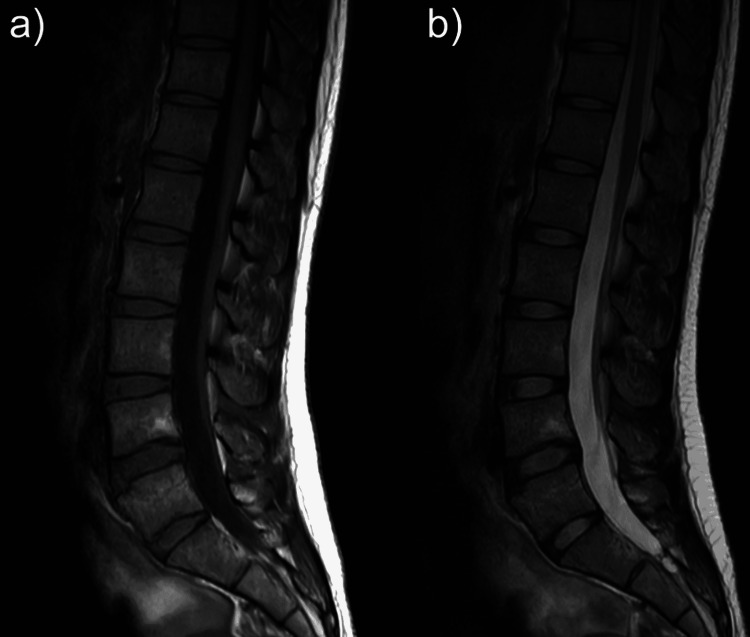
MRI at six months after onset (a) T1-weighted imaging (T1WI). (b) T2-weighted imaging (T2WI)
